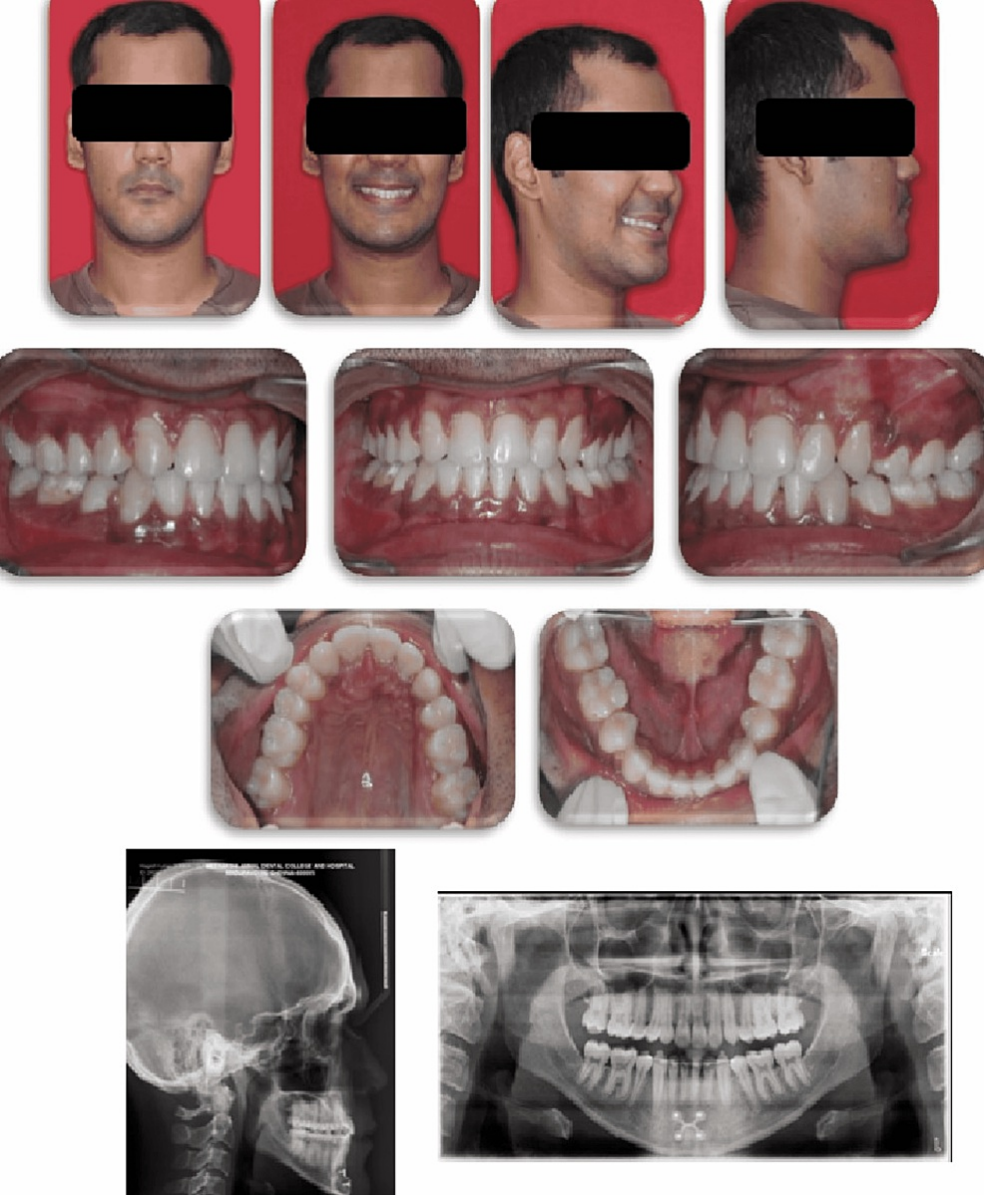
Post-treatment extraoral and intraoral photographs (lateral cephalogram and orthopantomogram)
